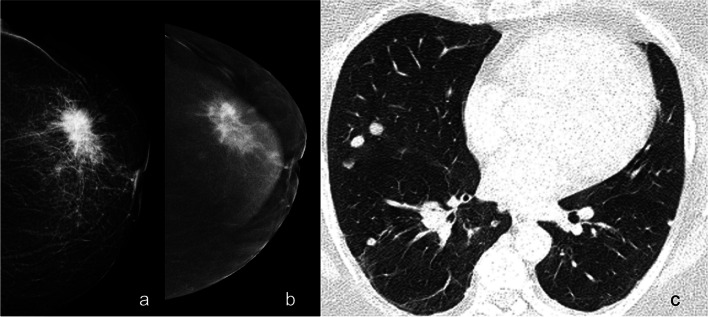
Breast cancer of the left breast appearing as a large spiculated mass on mammography (a). CEM correctly depicts the disease extent with involvement of retroareolar ducts (b). Thoracic CE-CT scans showing bilateral lung metastases (c). *CE-CT*, Contrast-enhanced computed tomography; *CEM*, Contrast-enhanced mammography
